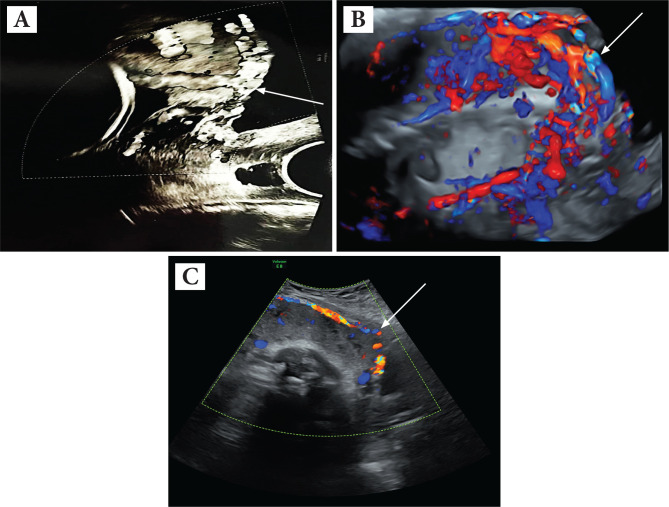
Ultrasound findings: (A) anterior placenta previa with abnormal lacunae, neovascularity, (B) bulge sign, no myometrium detectable, no clear zone, hypervascularity; (C) neovascularity with abnormal lacunae.
